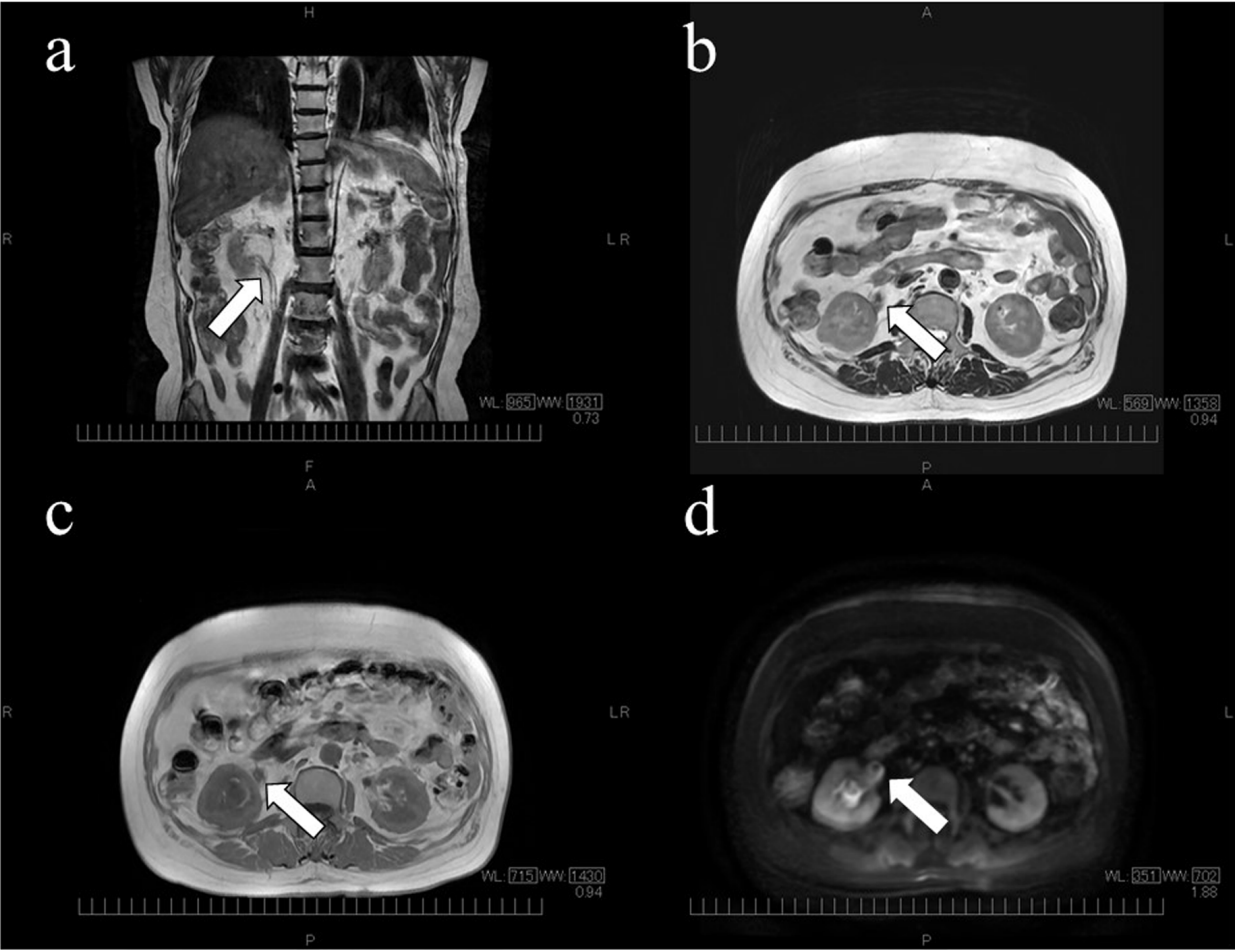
Nuclear magnetic resonance imaging on day 2. (a) T2-weighted coronal image, (b) T2-weighted axial image, (c) T1-weighted axial image, (d) Diffusion-weighted image The right renal pelvic ureteral transition area was suspected of inflammatory lesions and debris (arrow)
